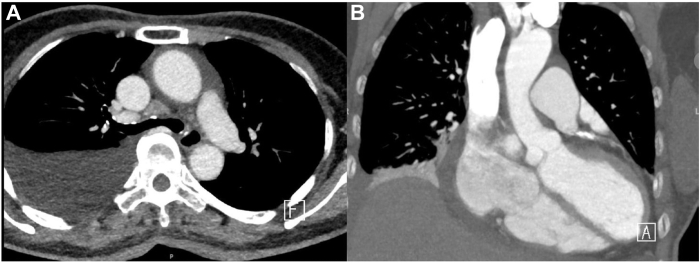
Axial (A) and coronal (B) chest computed tomography images obtained 2 weeks after cryoablation reveal an elevated right diaphragm and pleural effusion.
